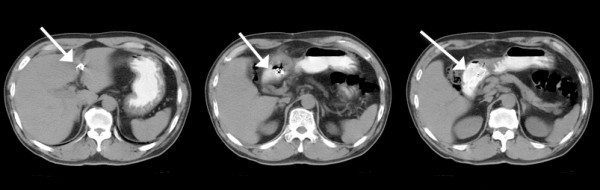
Abdominal CT scan. The CT-scan reveals transmural migration of the gauze into the duodenum (white arrow).
